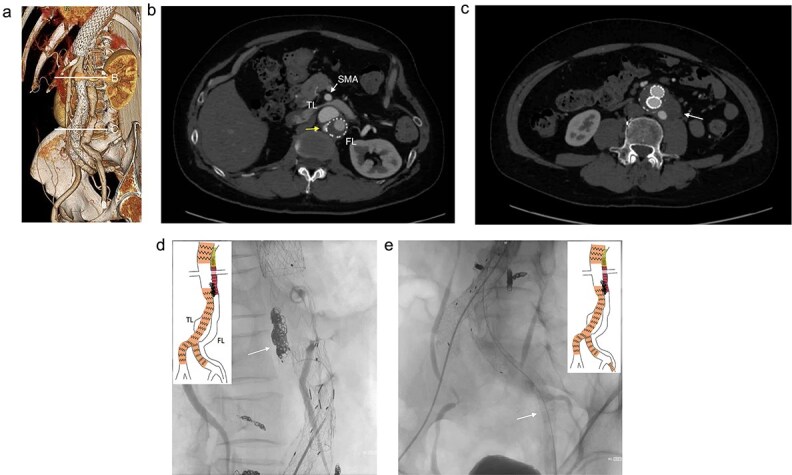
The contrasted CT 3D reconstruction 2 years after the false lumen (FL) stent graft placement (a; white arrow); the contrast-enhanced CT axial images showing small inflow to the FL from the true lumen through the gap between the intimal tear and the FL stent graft (b; arrow), and abdominal aortic aneurysm expansion to 56 mm (c; white arrow); the intraoperative angiography showing coiling of the gap between the intimal tear and FL stent graft (d; white arrow), and the VBX was deployed at left external iliac artery covering the re-entry tear (e; white arrow). TL: true lumen; FL: false lumen; SMA: superior mesenteric artery.
